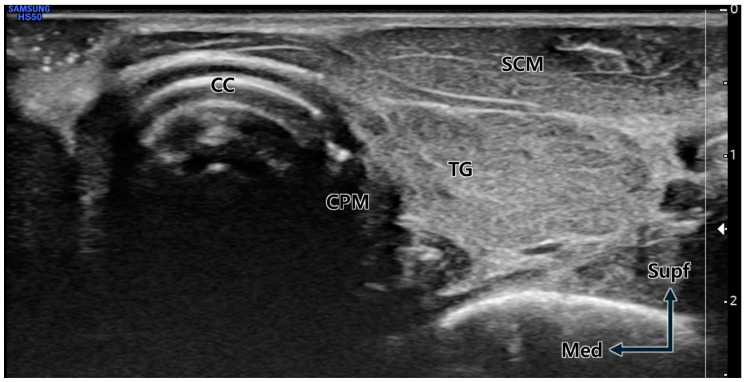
Transverse ultrasound image at the cricoid cartilage (CC) level. Due to the neck rotation to the lateral side, the cricopharyngeus muscle (CPM) is prominently visible immediately lateral (posterior) to the distinctly observed CC. TG; thyroid gland, SCM; sternocleidomastoid muscle, Supf; superficial, Med; medial.
